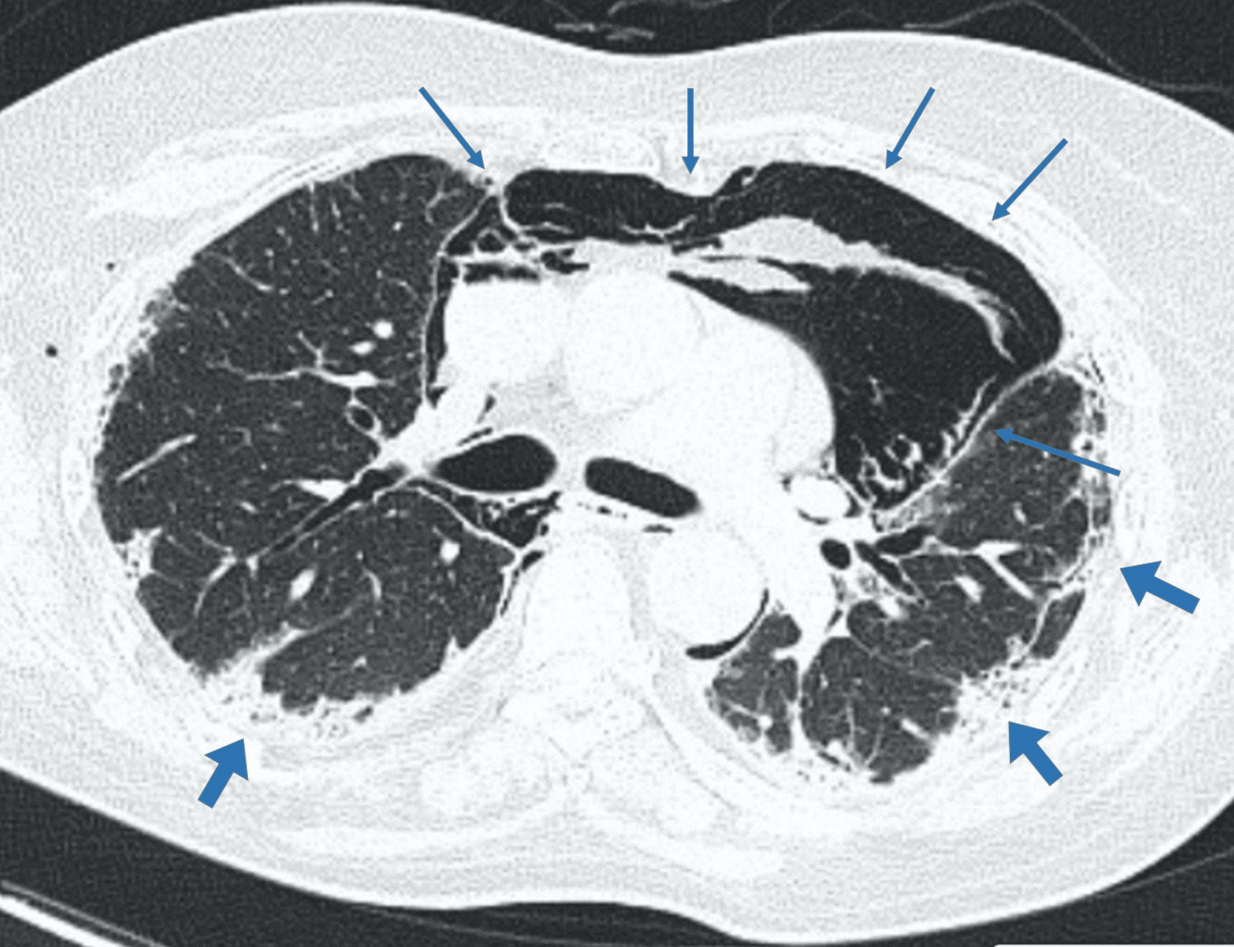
Initial chest CT scan Chest CT image showing an extensive pneumomediastinum (thin arrows) and some small areas of subcutaneous emphysema. The lung parenchyma presented thickening of the interlobular septa alongside bronchiectasis with a peripheral and basal predominance. Some of these areas were surrounded by ground-glass opacities and consolidations (thick arrows).
